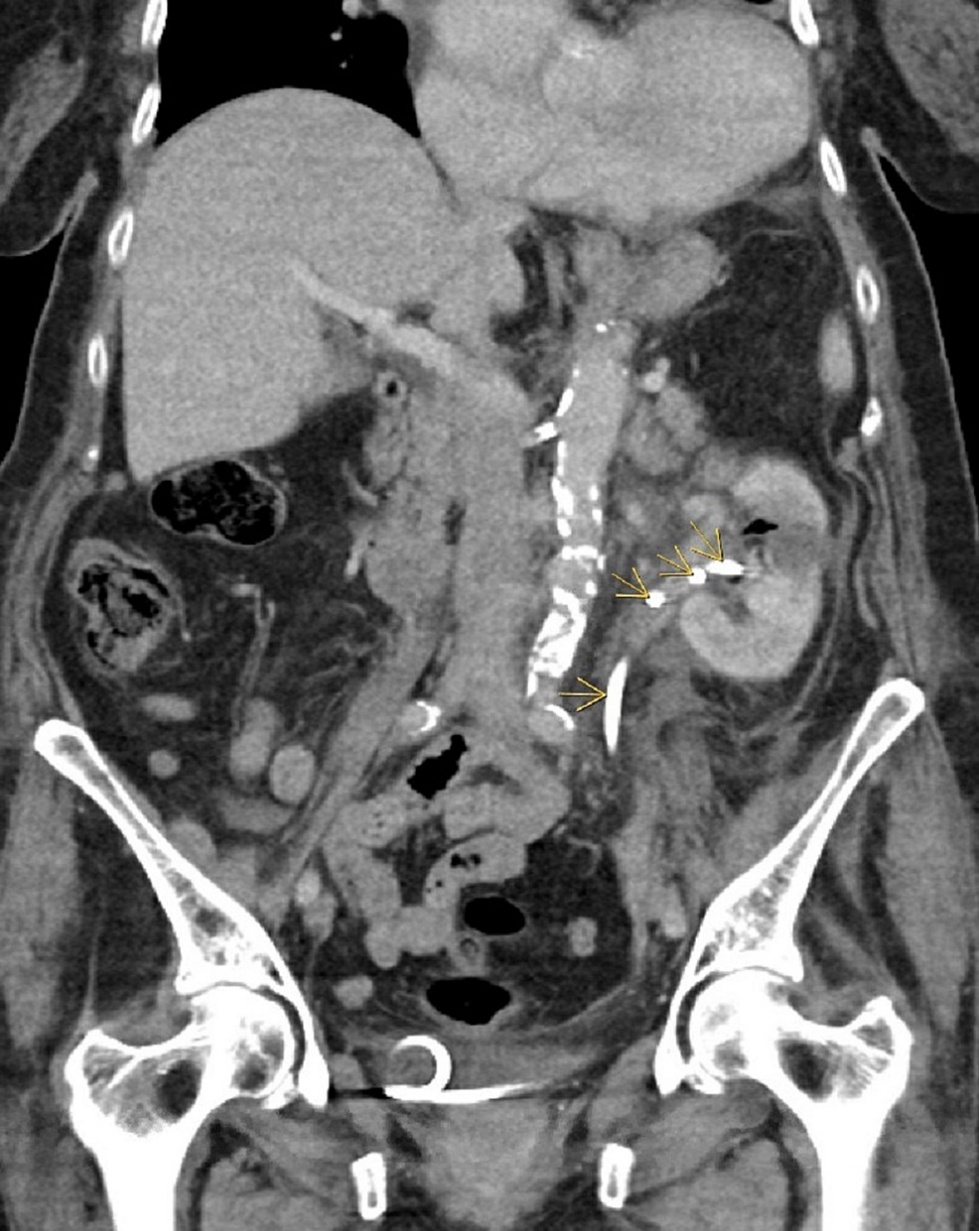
CT abdomen and pelvis coronal view showing PNCT (yellow arrows). PNCT - percutaneous nephroureteral catheter tube
